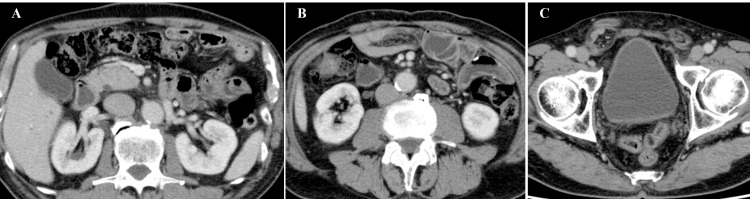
Abdominal CT taken after ACT. (A) The omentum, which is a fatty, viscous tissue located in the abdominal cavity, was no longer visible. (B) Ascites resulting from the dissemination of duodenal cancer had resolved. (C) Right inguinal hernia formed by abdominal pressure caused by massive ascites persisted. ACT, adoptive cell therapy.
